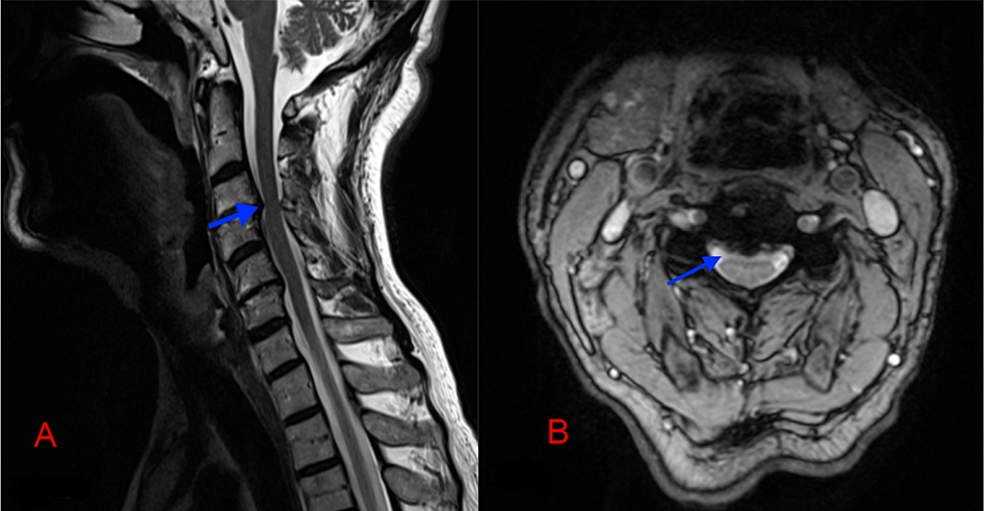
(A) Preoperative MRI T2-sagittal and (B) T2-axial sequences, blue arrows show the C3-4 spine canal stenosis.
